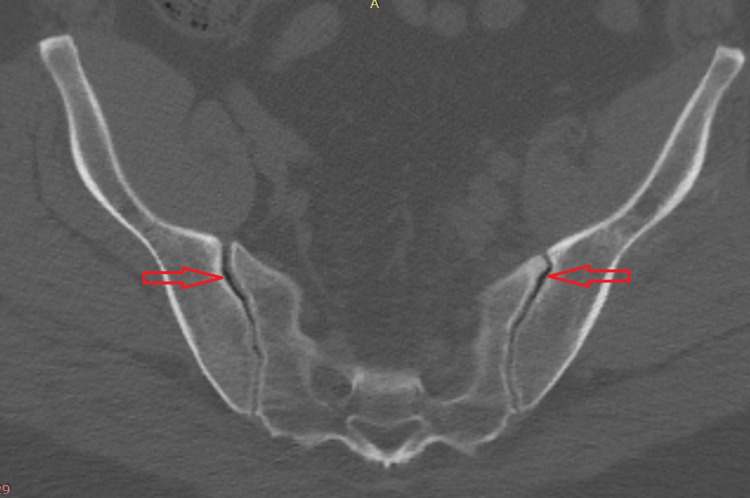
Preoperative CT scan showing bilateral SIJ vacuum sign CT: computed tomography; SIJ: sacroiliac joint
